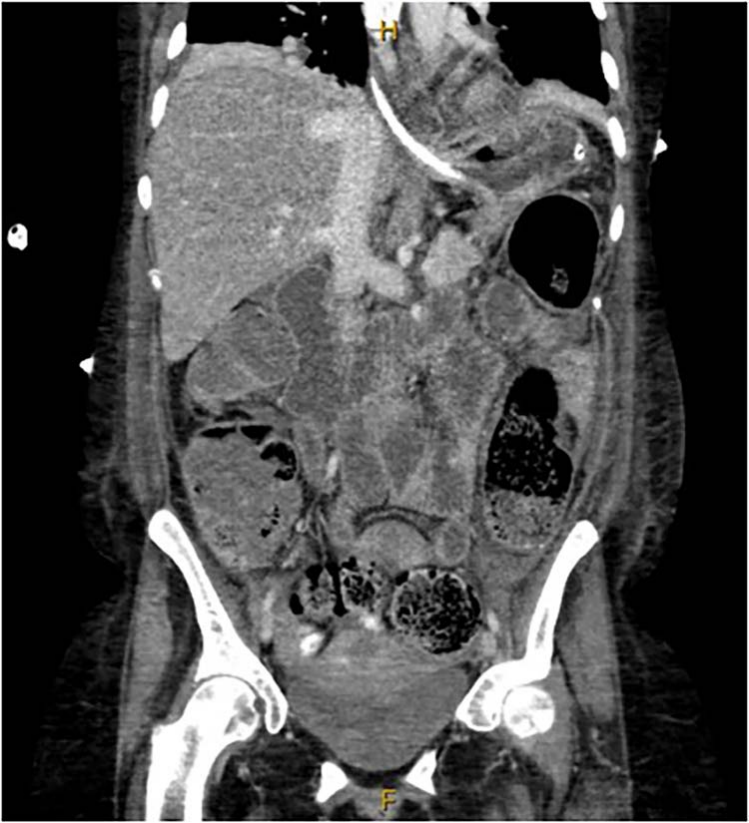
Coronal view of CT abdomen demonstrating normal large bowel wall enhancement with faecal loading.
